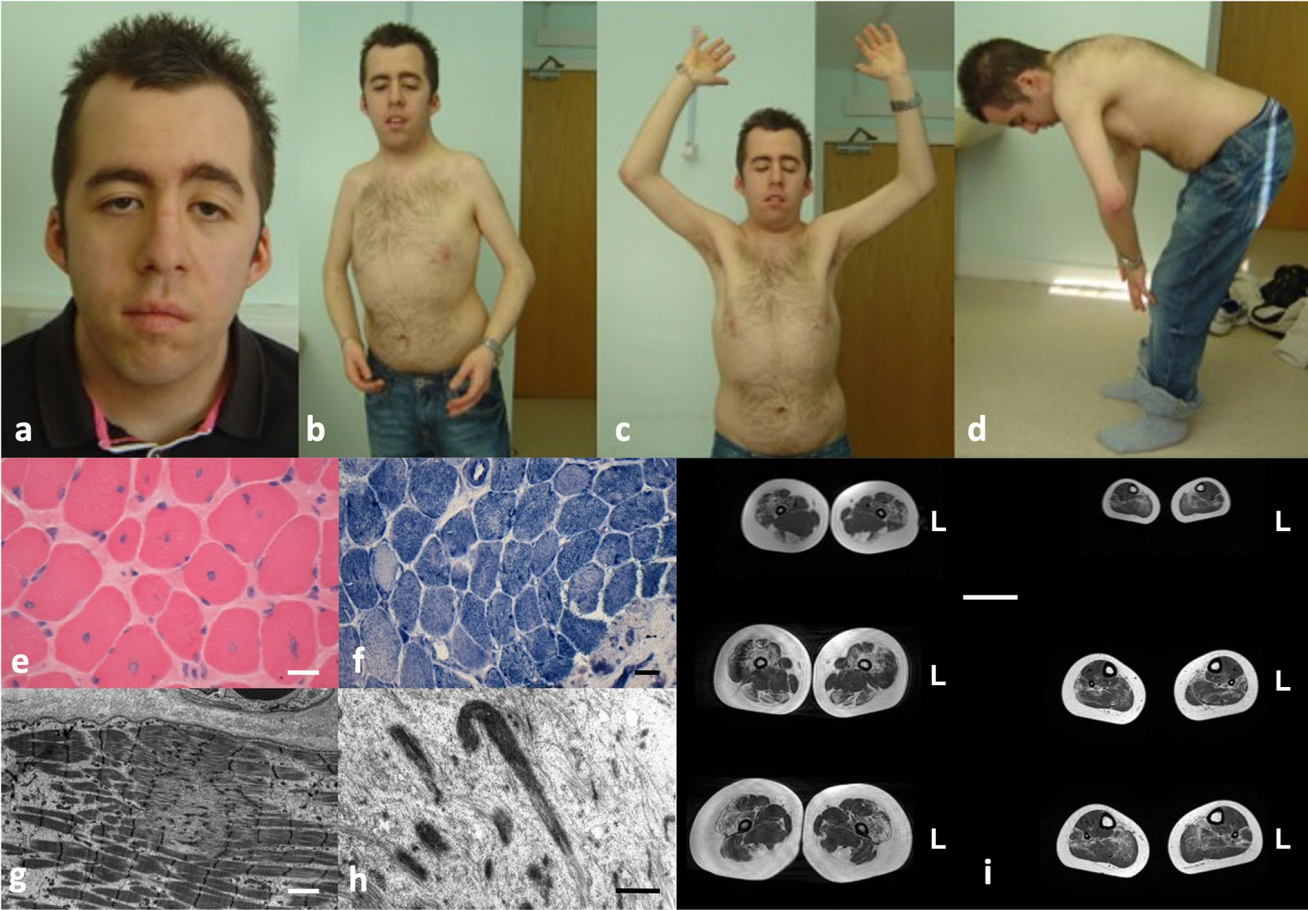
Typical features in *TTN*-related myopathies. This 38-year-old patient presented with hypotonia and ventricular non-compaction from birth. Subsequently, his motor development was delayed. His stature was short, he had a myopathic face with pronounced ptosis (a), multiple contractures prominently involving the elbows and shoulders (b, c), and spinal rigidity (d). He developed a dilated cardiomyopathy from his teens. The most prominent feature on muscle biopsy are numerous centralized nuclei (e) leading to an initial diagnosis of CNM, but there were additional cores (f, g) and few nemaline rods (h) on EM. Scale bars 40 µm (e, f), 2 µm (g) and 1 µm (h). Lower extremity muscle MRI from another patient showing prominent hamstring involvement in the thigh (i). Scale bar 5 cm, L indicates left side
